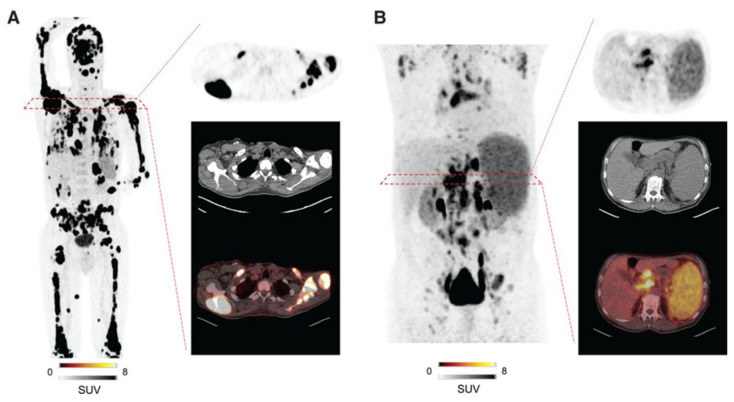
Maximum-intensity projections of patients with hematologic malignancies imaged with CXCR4-directed ^68^Ga-Pentixafor. Target lesion is also displayed on transaxial PET, CT, and PET/CT. Patient diagnosed with multiple myeloma (A; SUVmax in target lesion, 74.3) and MCL (B; SUVmax in target lesion, 17.2). Substantially low background activity allowed for precise determination of disease sites. (Figure from [111]. Copyright © Society of Nuclear Medicine and Molecular Imaging).
